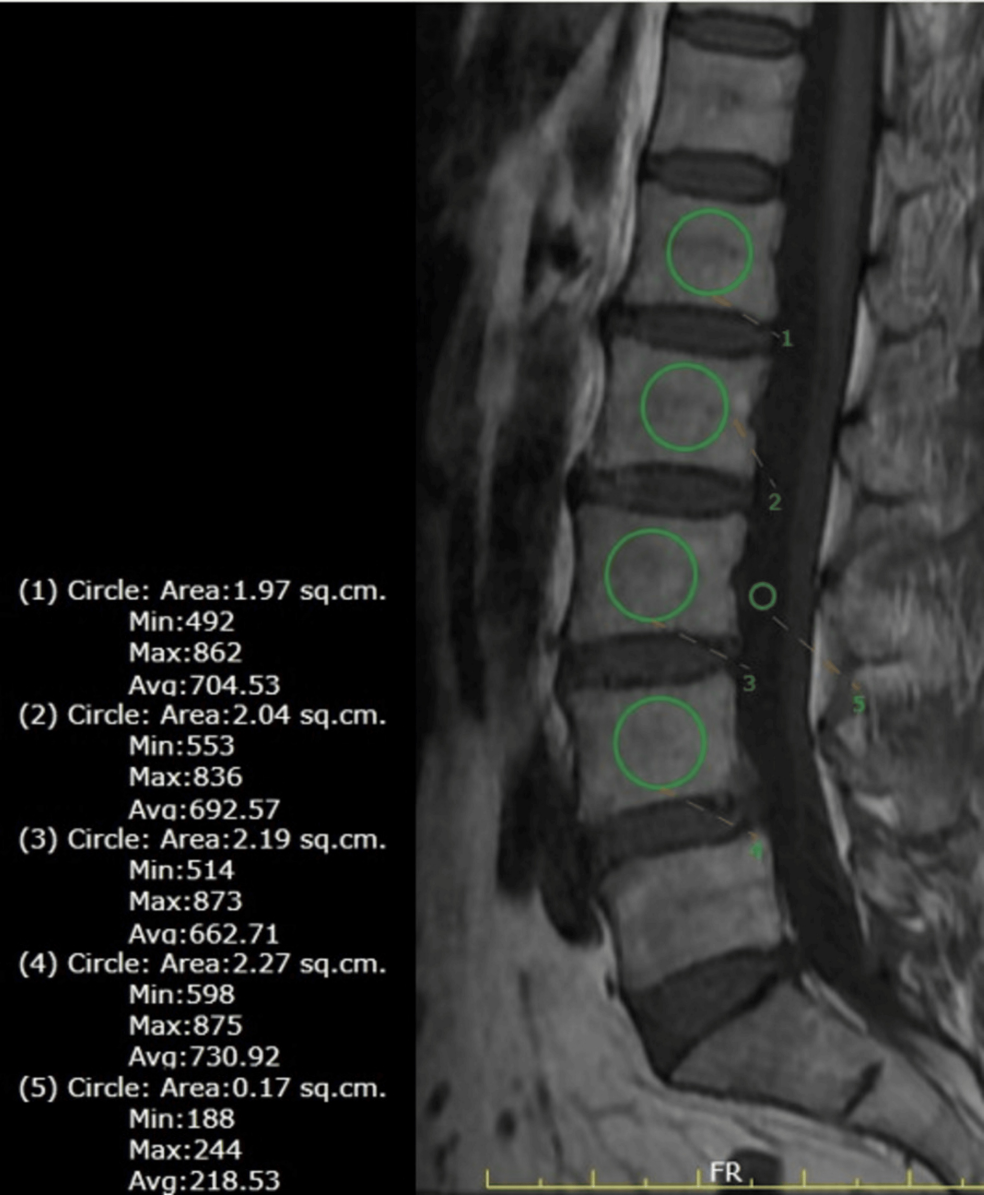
T1-weighted mid-sagittal image showing equal-sized ROIs drawn within the L1-L4 vertebral body and another ROI drawn within the anterior subarachnoid CSF space at the L2 level. ROI: region of interest, CSF: cerebrospinal fluid.
